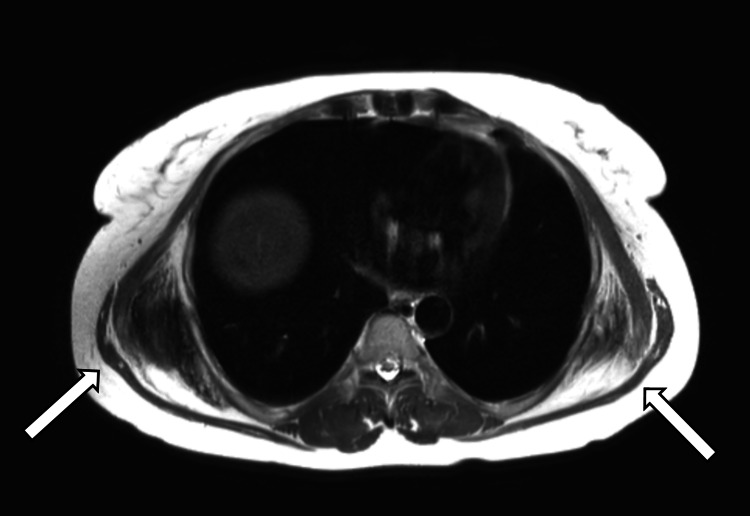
T2-weighted MRI sequence (axial view) without fat saturation on May 27, 2024 bilateral soft-tissue masses located deep to the serratus anterior muscles, displaying intermediate signal intensity with interspersed linear hyperintensities corresponding to internal fat strands (arrows), consistent with elastofibromas.
